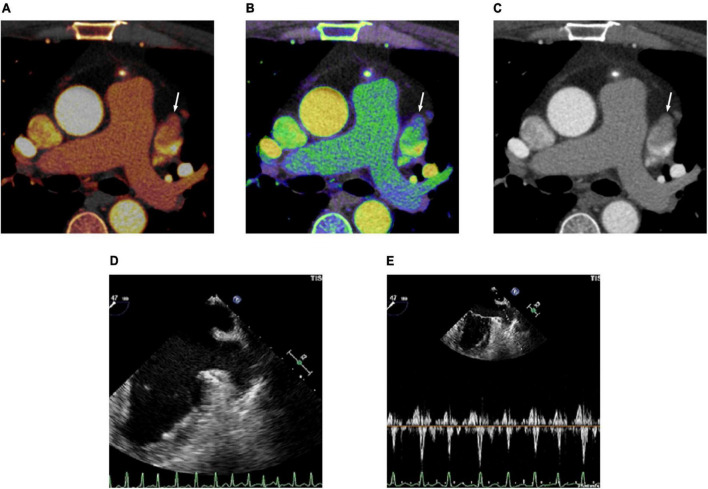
Cardiac computed tomography (CT) and transesophageal echocardiography (TEE) images in 48-year-old man with spontaneous echo contrast (SEC). (A) Dual-energy CT-derived iodine map shows filling defect in the left atrial appendage (LAA) (arrow) with LAA/AA iodine concentration ratio of 0.13 (true negative finding). (B) Dual-energy CT-derived Z_*eff*_ image shows filling defect in the LAA (arrow) with LAA/AA Z_*eff*_ ratio of 0.68 (true negative finding). (C) Conventional CT image shows filling defect (arrow) in the LAA with LAA/AA HU ratio of 0.24 (false positive finding). (D) TEE image demonstrates SEC in LAA. (E) Doppler flow measurement of TEE shows reduced emptying velocity of 37 cm/s but measurable flow throughout the LAA.
